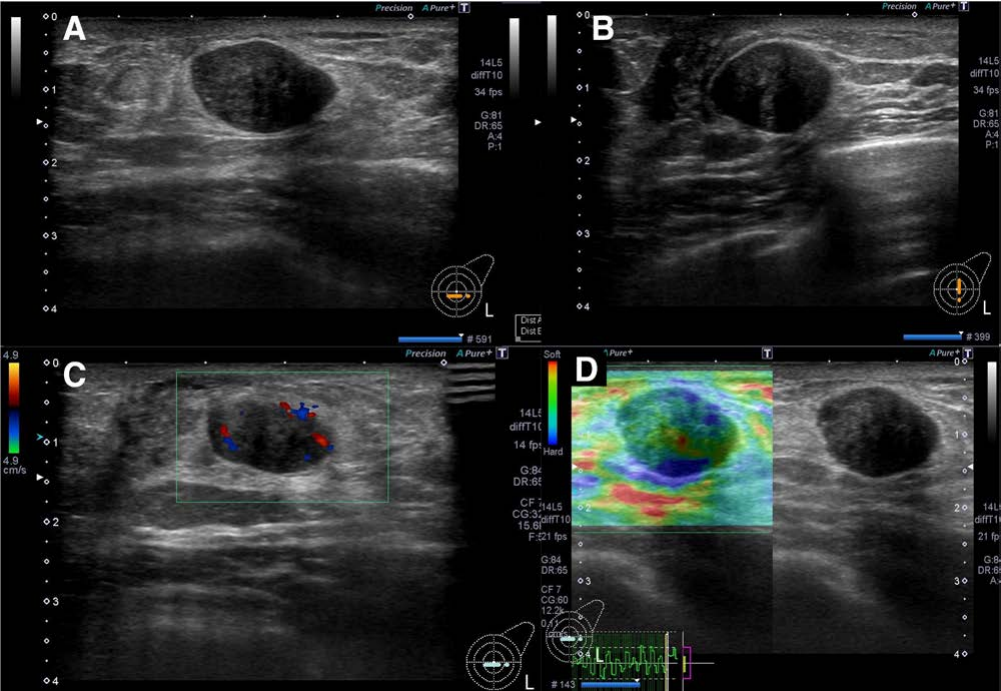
Breast ultrasound showing (a, b) an oval, well-circumscribed and hypoechoic mass measuring 20.8 × 17.8 × 12.4 mm with an anteroposterior/longitudinal ratio of 0.60. (c) Color doppler ultrasound reveals some central and peripheral color flow signals. (d) The strain elastography shows an overall intermediate hardness colored in green, consisting of heterogeneous hard and soft zone colored in blue or red.
